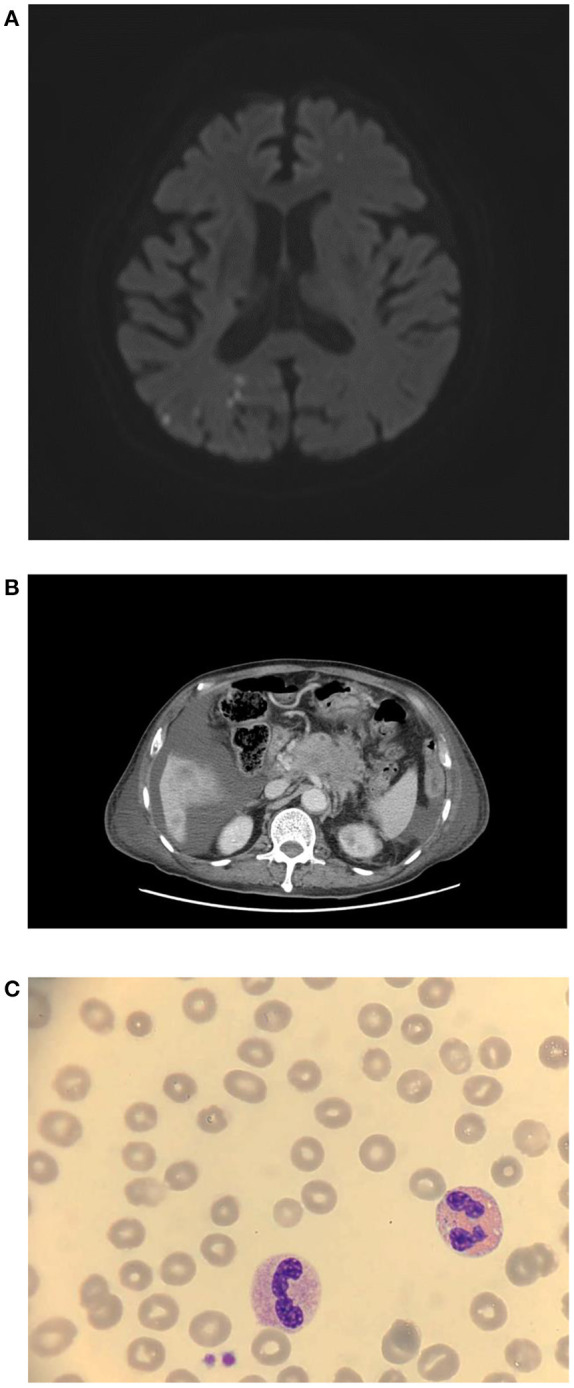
(A) Brain MRA revealed diffuse right posterior cerebral territory infarction, 7 days after vaccination. (B) Accidentally, stage IV pancreatic cancer was found on abdominal CT. (C) Blood smear, 9 days after vaccination.
